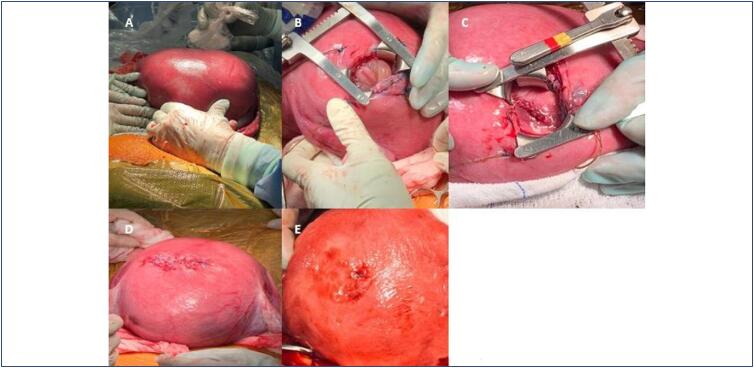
(A) Exteriorization of the uterus with ultrasound mapping of placental insertion and identification of fetal position. (B) Exposure of the fetal myelomeningocele by hysterotomy with an Ankeney retractor. (C) Repair of the myelomeningocele by the neurosurgical team. (D) Hysterorrhaphy with identification of the suture in the uterine serosa. (E) Identification of the uterine scar from myelomeningocele repair after cesarean section
